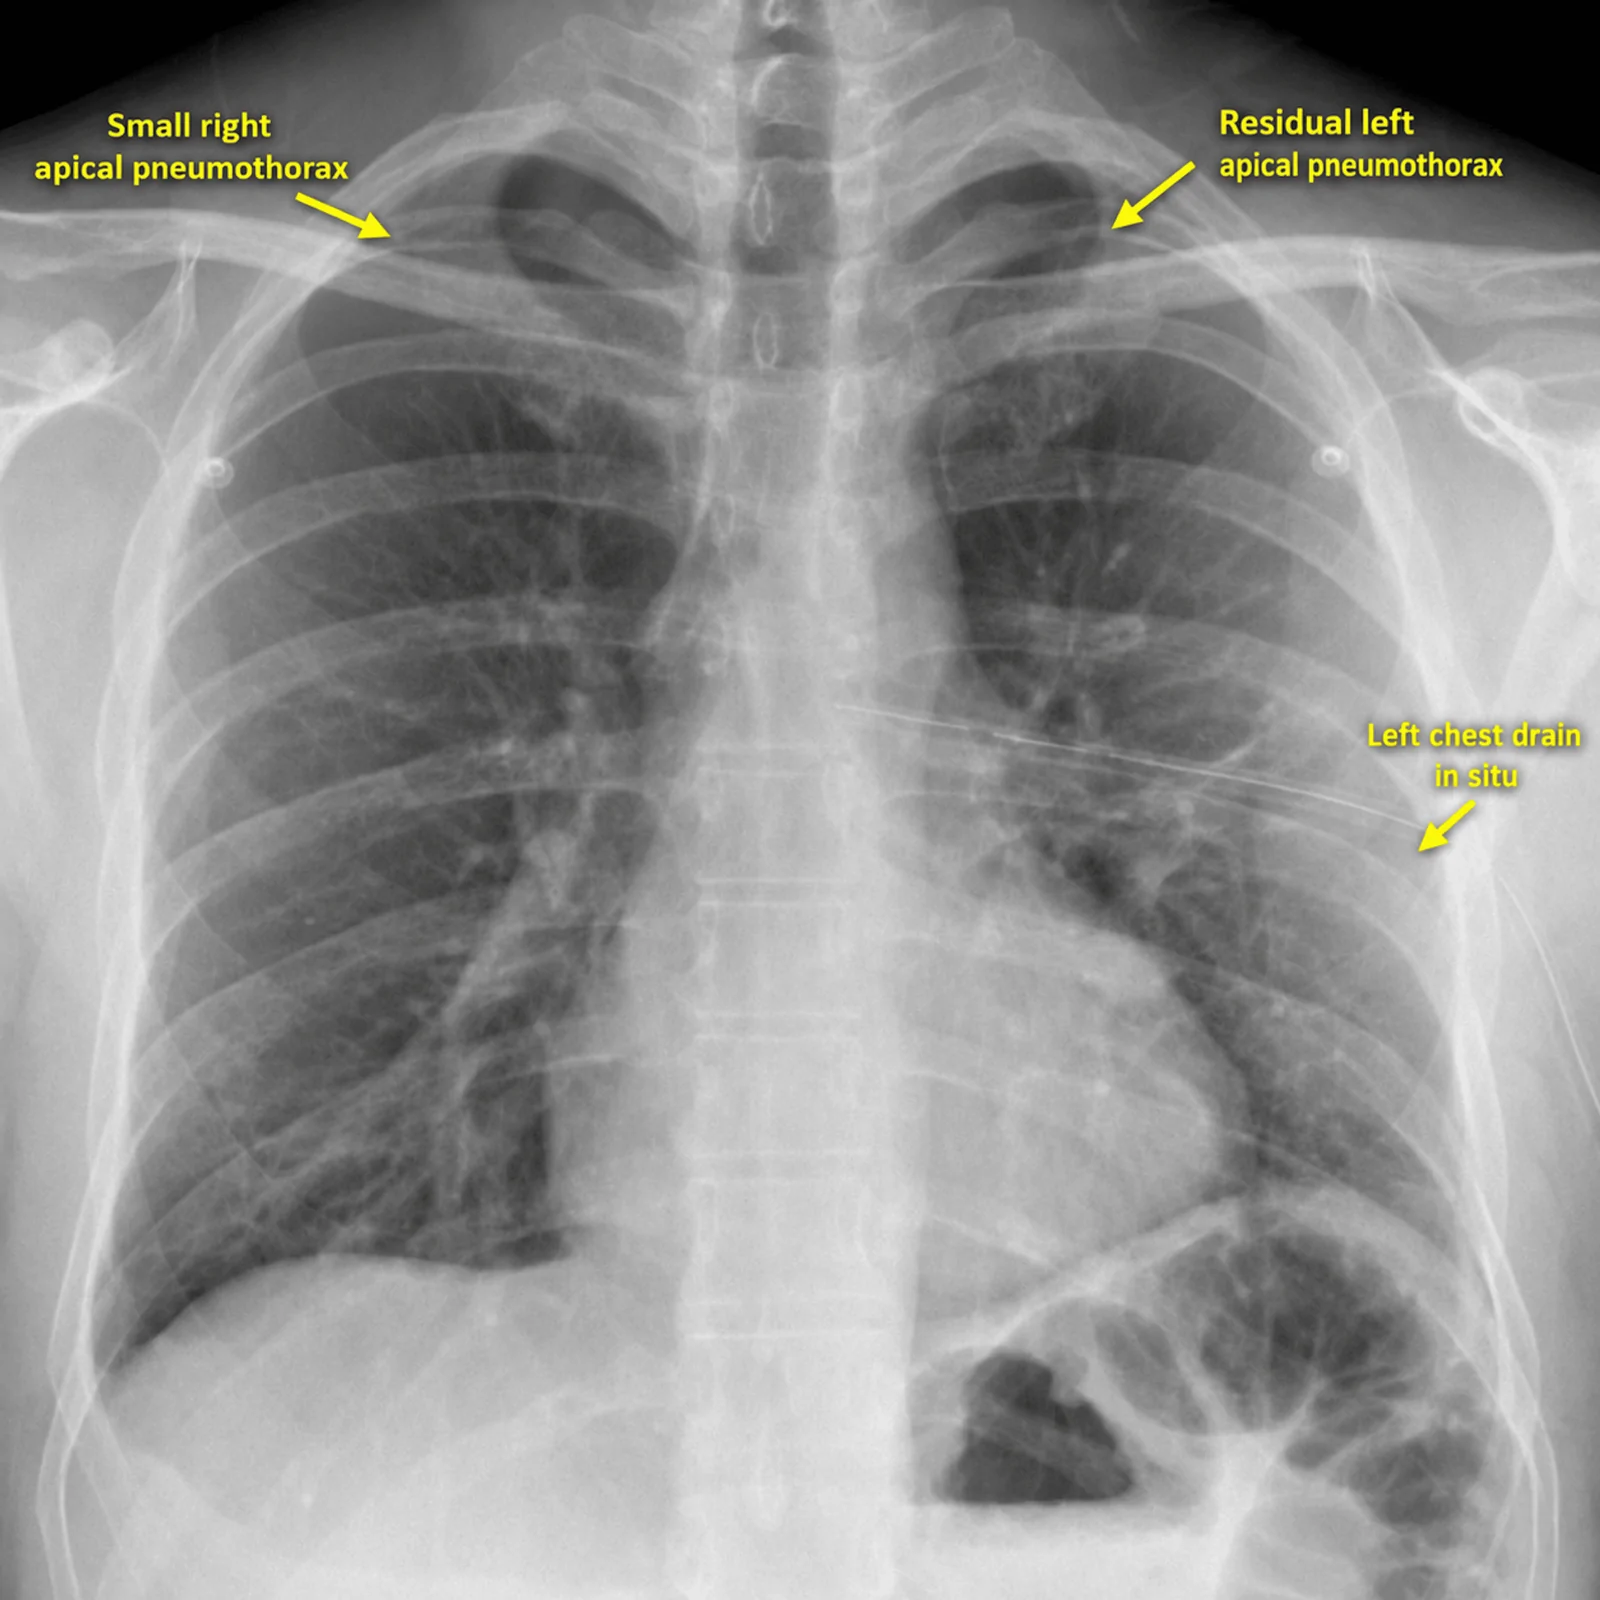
Separate follow-up chest radiograph: small right apical pneumothorax identified after treatment of the dominant left-sided pneumothorax The annotation identifies the right apical pleural line.
